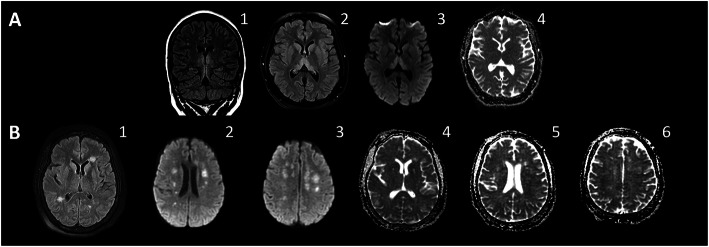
Para-infectious autoimmunity. a Acute necrotizing encephalopathy (ANE). A 23-year-old SARS-CoV-2-positive female presented mainly with encephalopathy progressed to catatonia. C-reactive protein (CRP) was 72.3 mg/dl. CSF analysis was completely normal. Coronal (1) and axial (2) fluid-attenuated inversion recovery (FLAIR)/T2-weighted (T2W) MR images reveal signal hyperintensities of the ventromedial thalami and hippocampi. DWI (3) and ADC (4) images show slightly increased signal intensity on DWI but without low signal intensity on ADC to suggest diffusion restriction. Bilateral thalamic involvement is highly characteristic for ANE. Her neurological condition improved substantially following immune therapies that included intravenous immunoglobulin, intravenous pulse methylprednisolone, and rituximab. b Acute disseminated encephalomyelitis (ADEM). A 56-year-old SARS-COV-2 positive female admitted for diarrhea and hypoxemic respiratory symptoms that progressed to ARDS necessitating intubation. Notable laboratory findings were CRP of 31 mg/dl (reference range 0.00–0.40), ferritin of 799 ng/ml (reference range 15–150 ng/ml), D-Dimer of 362 ng/ml (reference range < 230 ng/ml), and IL-6 of 42 (reference range less < 5 pg/ml). Neurological examination was notable for severe encephalopathy and severe quadriplegia. Axial FlAIR MR images (1, 2, 3) demonstrate multiple periventricular white matter hyperintensity lesions, without corpus callosal involvement. There is no enhancement or restricted diffusion on DWI (4, 5, 6). These findings are consistent with acute disseminated encephalomyelitis. Patient received a 5-day course of intravenous pulse methylprednisolone (1000 mg/day) and tocilizumab 750 mg (8 mg/kg) intravenously. She improved substantially to the point of becoming fully awake and alert with 4/5 power of upper extremities and 3–4/5 power of lower extremities. Patient was discharged to an acute rehabilitation facility and then went home showing a steady neurological improvement
